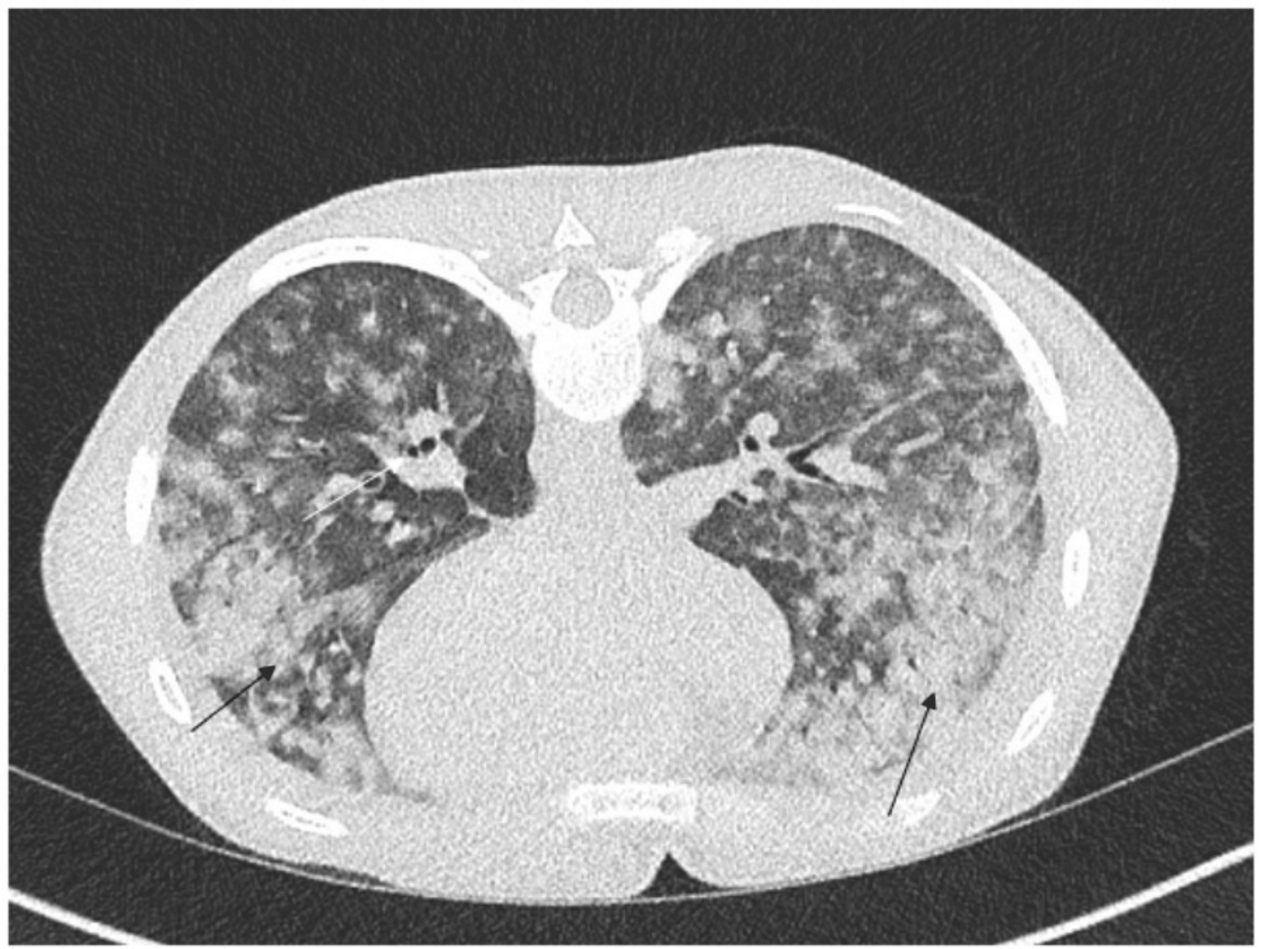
CT thorax of our patient showing diffuse consolidation with bronchiolar dilatation.
